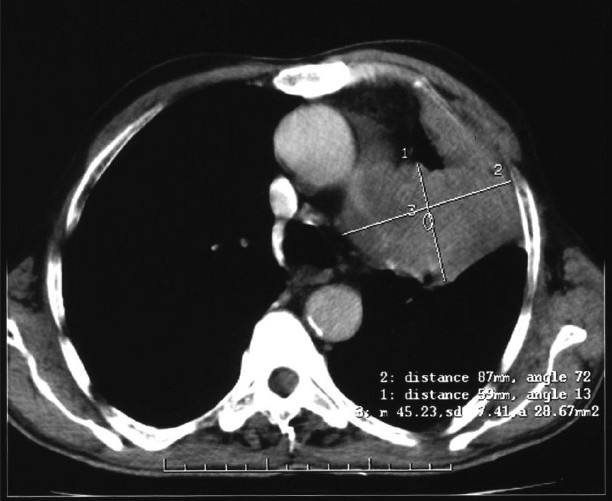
Chest CT scan showing left mid lobe mass
